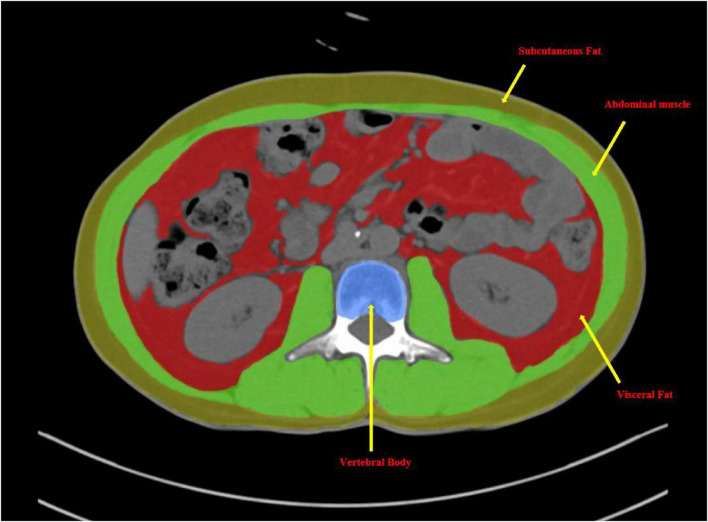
Representative axial abdominal computed tomography (CT) image at the L3 level with segmentation of body composition components. The yellow area indicates subcutaneous adipose tissue; the green area indicates abdominal skeletal muscle (including psoas major, erector spinae, quadratus lumborum, transversus abdominis, internal oblique, external oblique, and rectus abdominis); the red area indicates visceral adipose tissue; the blue area indicates the vertebral body. Yellow arrows highlight each color-coded component.
